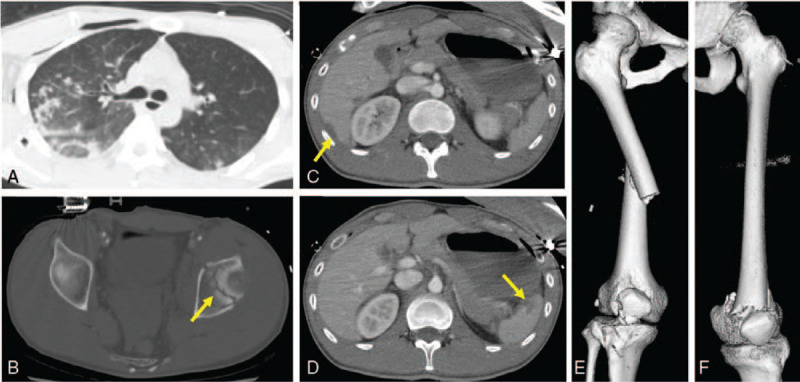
Computed tomography at the time of injury showing bilateral pneumothorax (A), left acetabular fracture (B), liver injury (C), spleen injury (D), right femoral fracture, patellar fracture, tibial plateau fracture (E), and left distal third femoral fracture (F).
